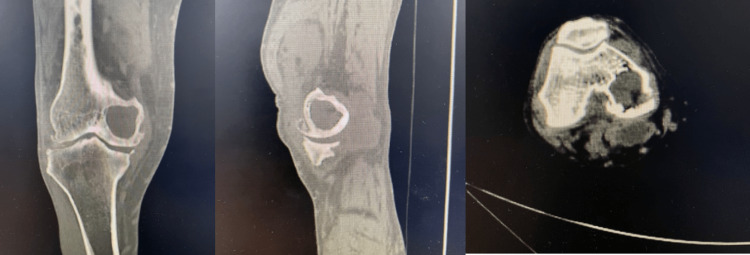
CT scan of the right knee showing bony cyst occupying medial femoral condyle.
